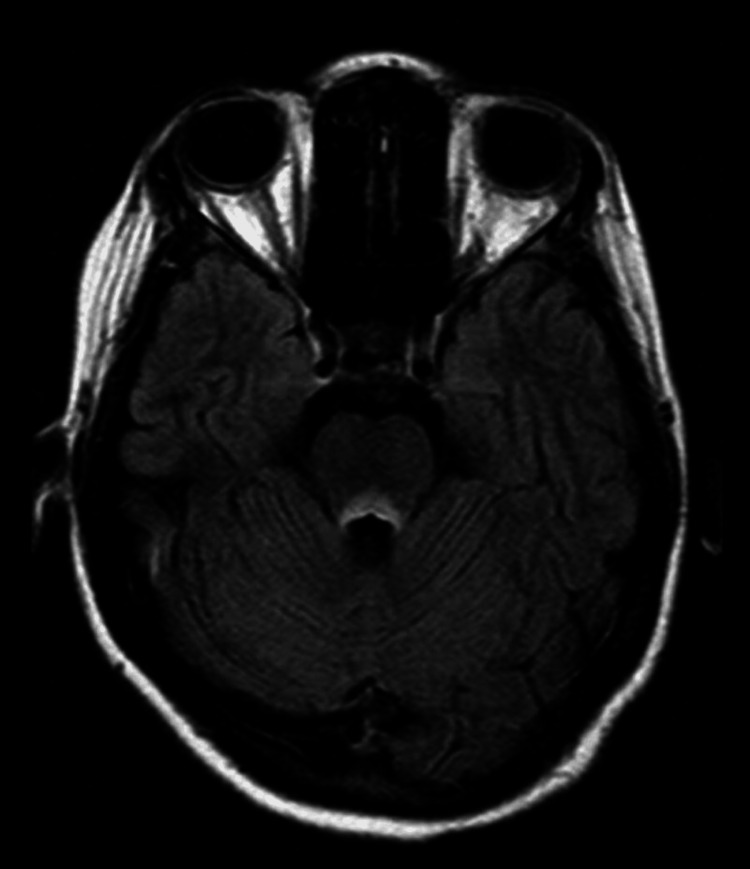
Axial T2-FLAIR MRI showed areas of symmetrical increased signal involving the mammillary bodies, dorsomedial thalami, tectal plate, periaqueductal area and/or around the third ventricle. FLAIR: fluid-attenuated inversion recovery
